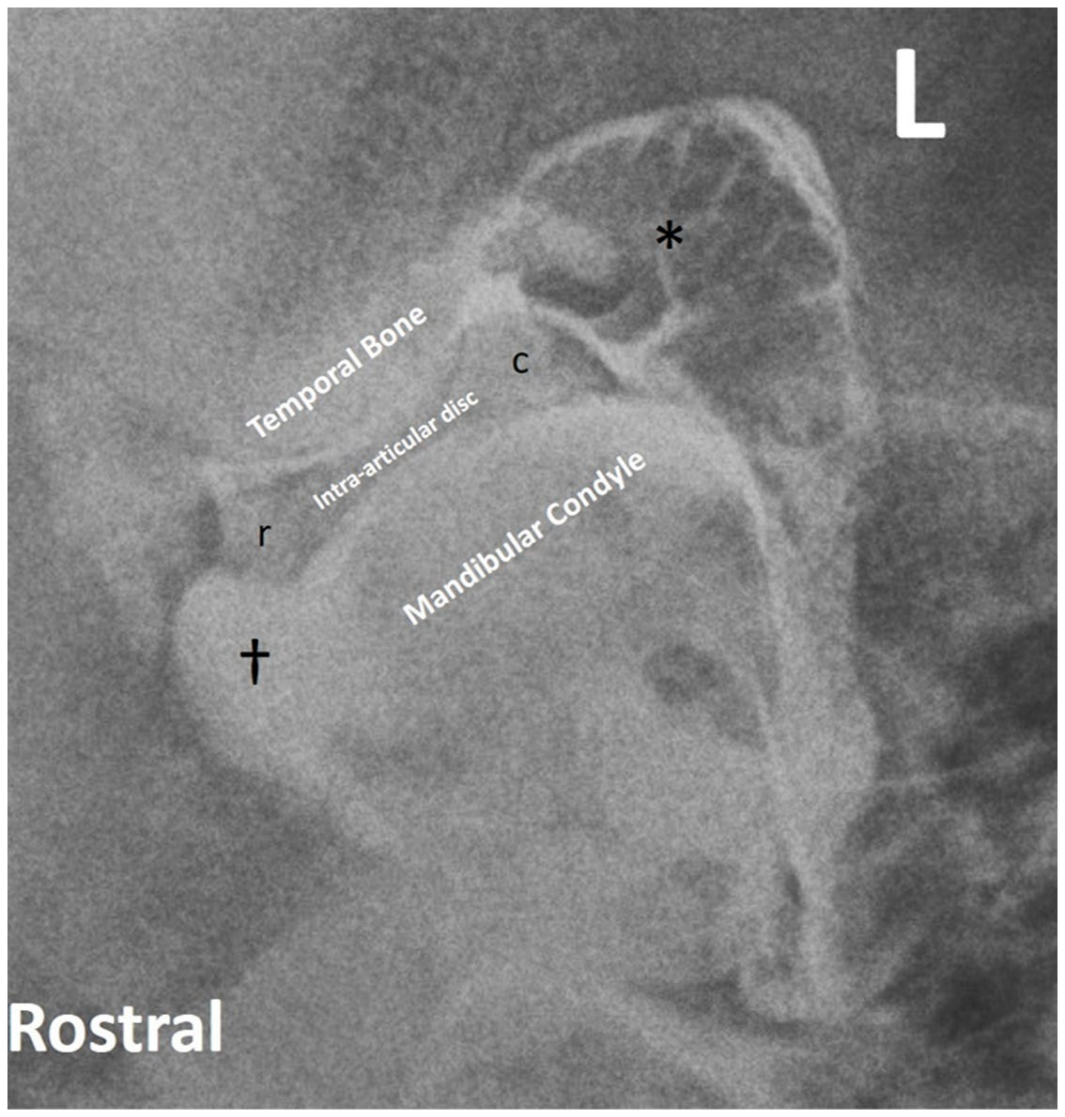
Double contrast lateral oblique radiographic projection of the left TMJ delineating joint margins (*), rostral (r) and caudal (c) disc, and contrast pooling (†).
